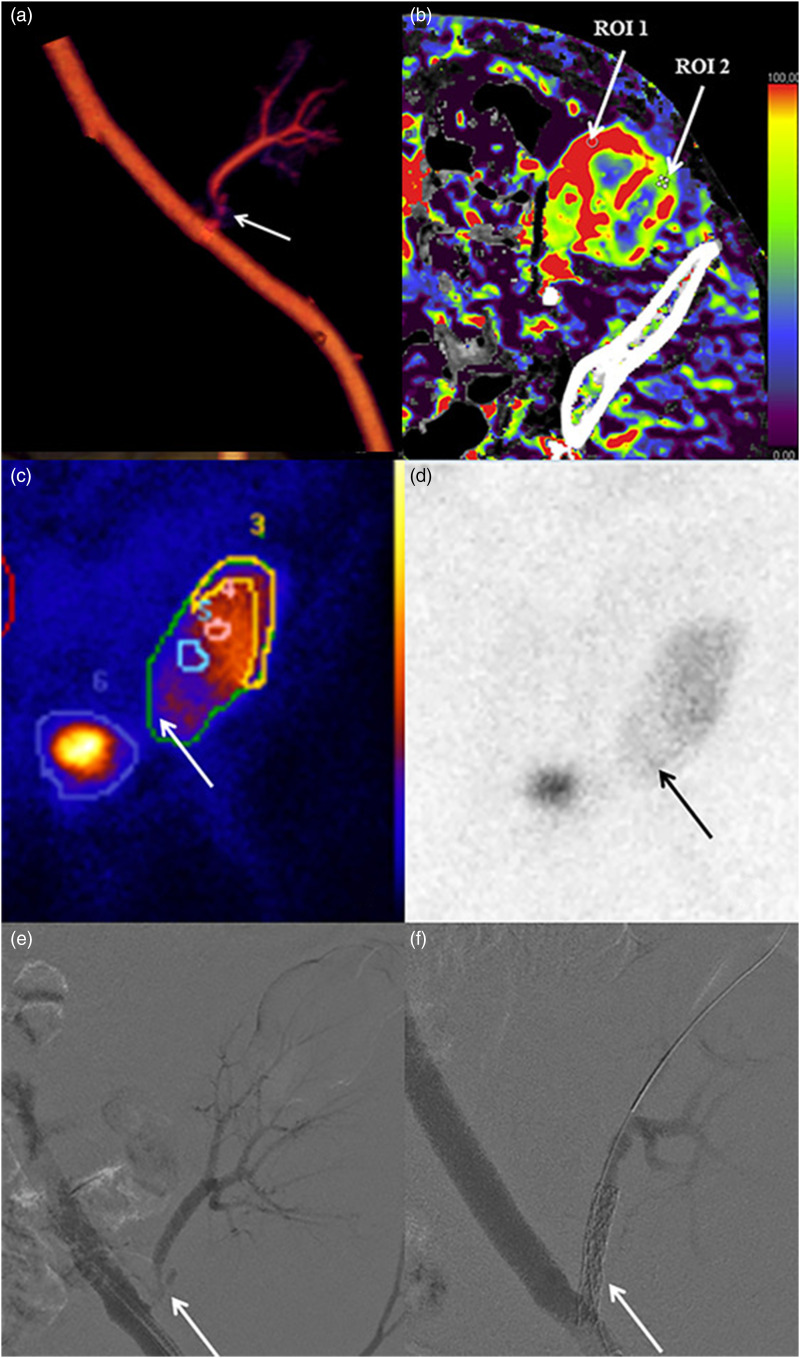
(a–f). Volume rendered CT image (a) showing stenosis of the renal artery. Color-coded CT perfusion (b) using the same data set acquired from the P-CTA showing decreased perfusion of the caudal renal cortex (ROI 2), perfusion defect confirmed by scintigraphy (c, d). Patient was referred for digital subtraction angiography (DSA) and angioplasty (e, f).
